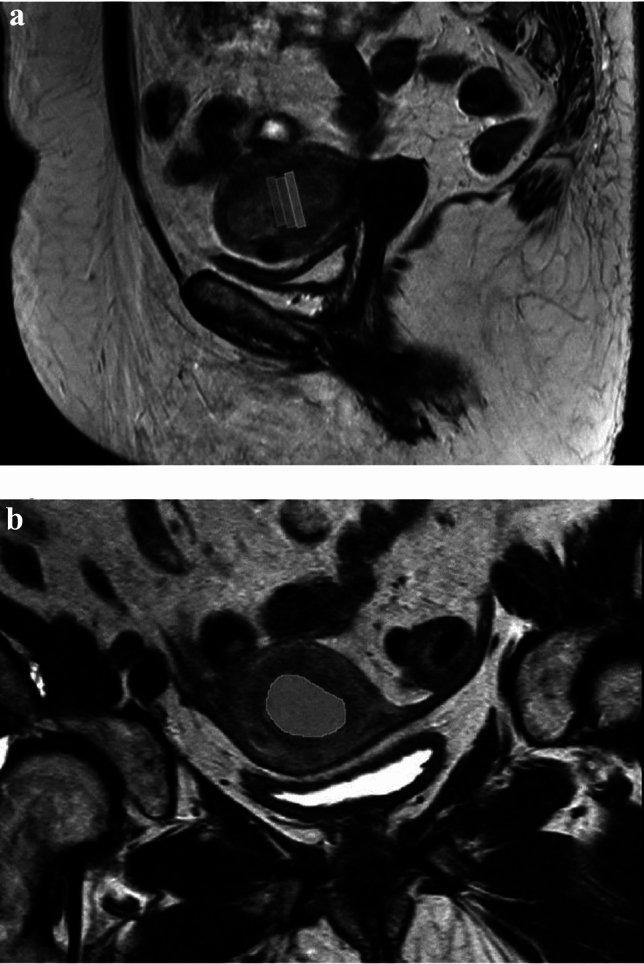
Segmentation on three neighbouring slices. a Sagittal T2-weighted image b Oblique T2-weighted image axial to the uterus
